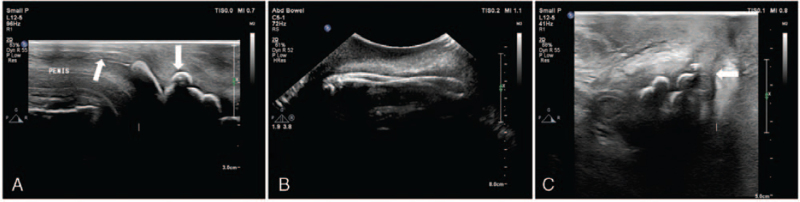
Ultrasonography features before surgical treatment. A. The high-frequency probe shows the posterior urethra and the arc strong echoic spot with shadowing in the middle urethra (arrows). B. Low-frequency probe shows the full view of the foreign body. C. The high-frequency probe revealed the damage of the central urethra (arrow) and urine leakage into the scrotal wall.
